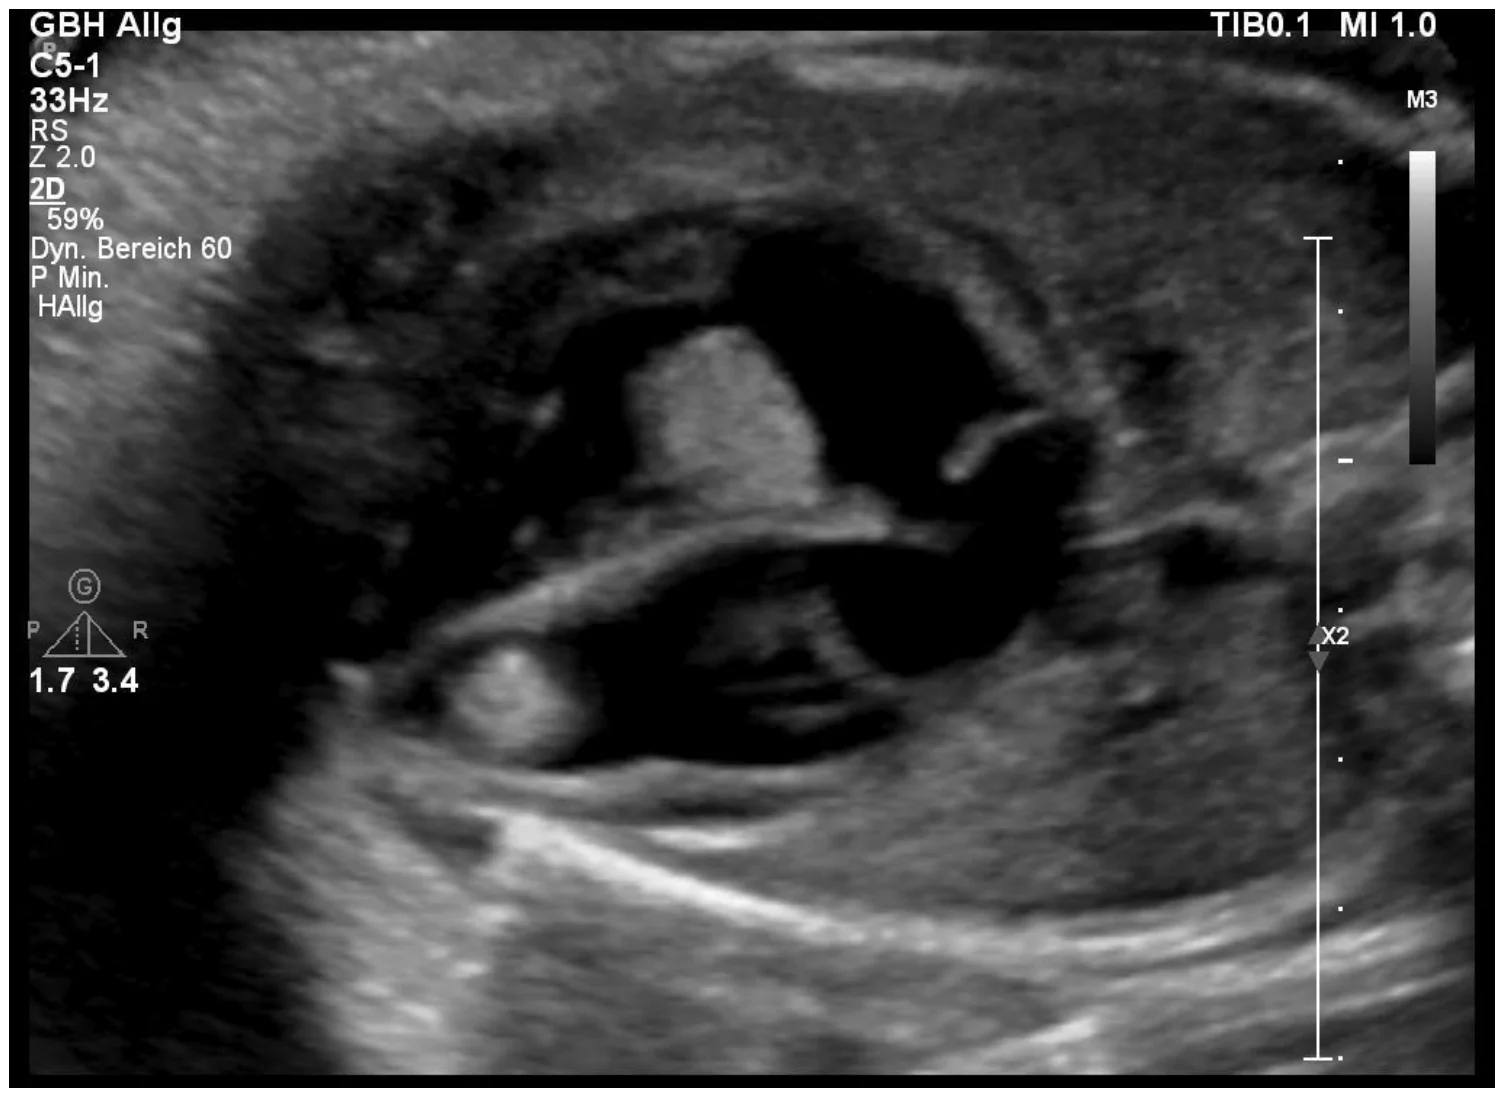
Fetal echocardiography demonstrates two large cardiac rhabdomyomas causing partial obstruction of the ventricular outflow tracts.
